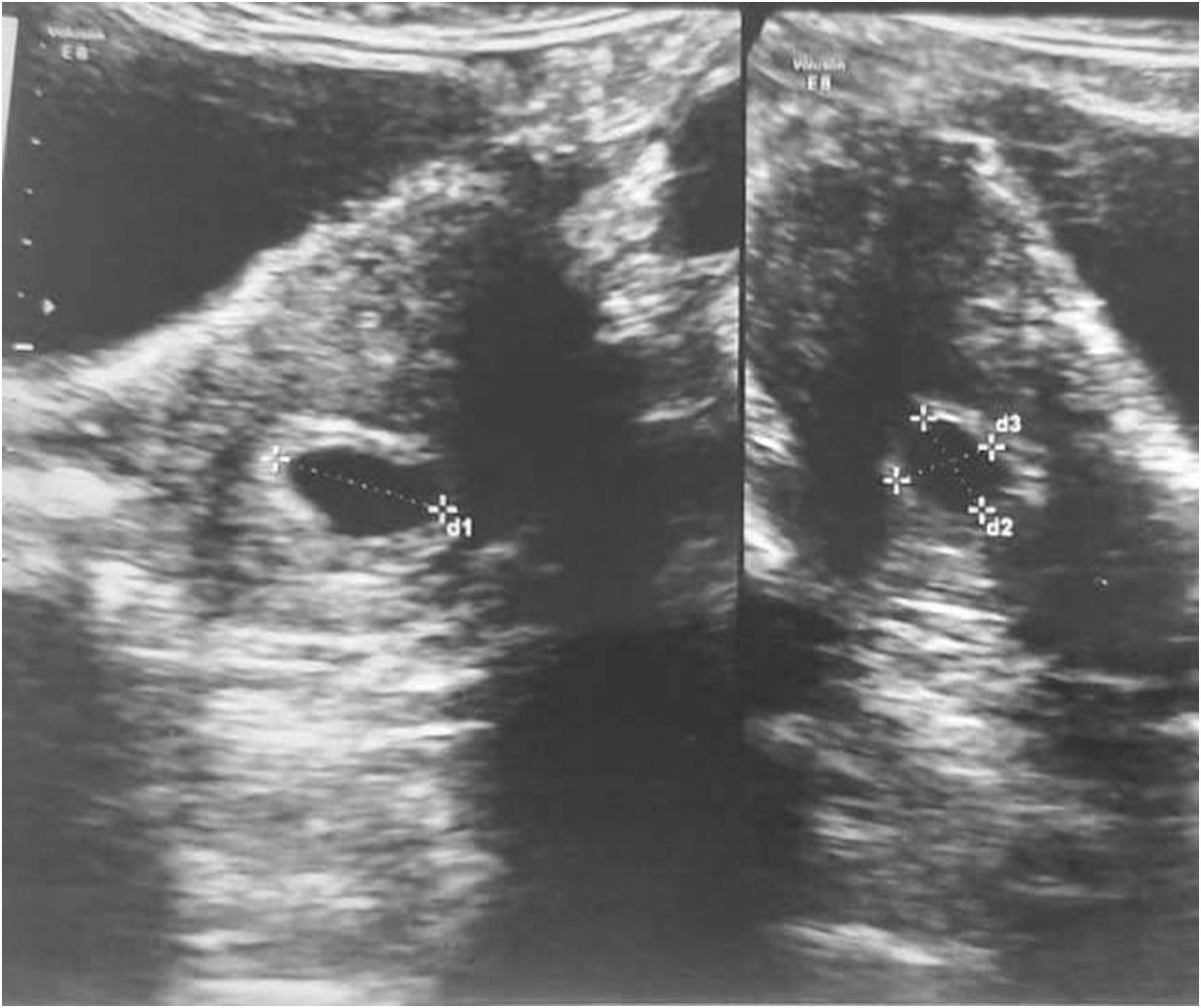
This abdominal ultrasonography shows a gestational sac containing a fetal pole.
